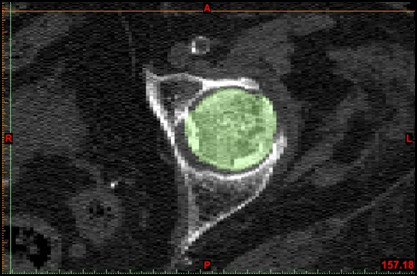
Medical Image Processing. Segmentation of bony tissue. (axial CT image of proximal left femur; bony tissue highlighted in color). [Mimics^© ^screenshot].
